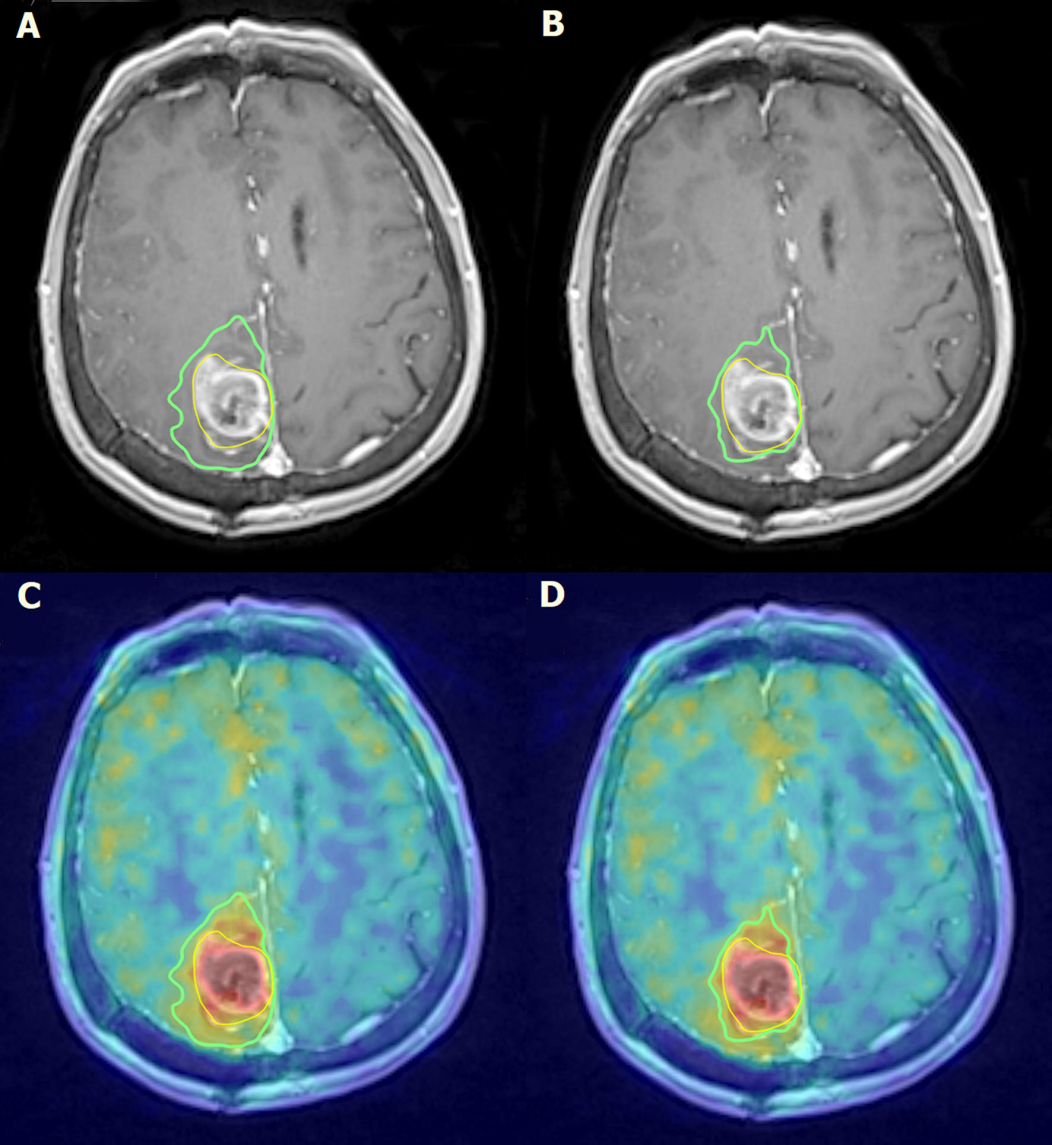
Parieto-medial postoperative status on the right side of the brain. In the surgical region, a fluid-containing cavity with small air bubbles and uneven contrast enhancement can be detected on post-contrast T1-weighted MRI images (A, B). Uneven 18F-FDOPA accumulation can be observed at the edges of the deviation on fused 18F-FDOPA PET/MRI images (C, D). Intense contrast and 18F-FDOPA accumulation suggests of the presence of a residual tumor around the surgical cavity. The yellow line describes the GTV volume (A–D), the green line on panels (A, C) describes the BTV 1.7 accumulation coverage and the green line on panels (B, D) describes the BTV 2.0 accumulation coverage.
